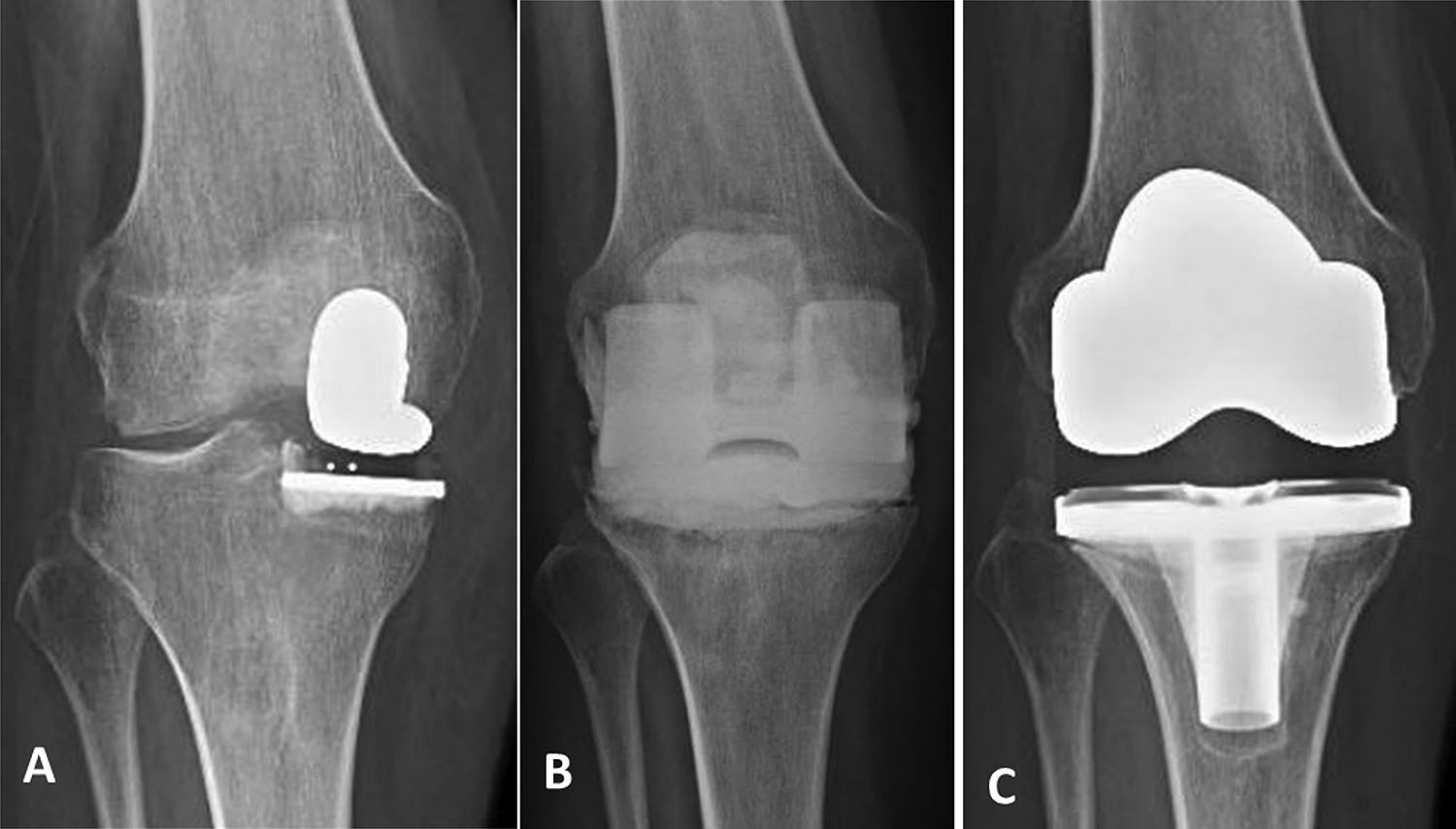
Radiological imaging of a UKA-infected patient. A Preoperative X-ray showing a right medial UKA. B Articulating spacer. C 4-year follow-up with a PS prosthesis implantation
